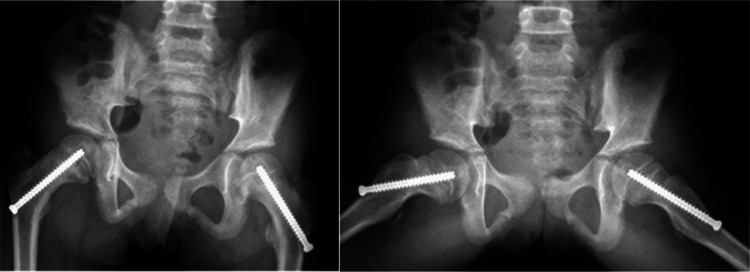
Anterioposterior and frog leg X-rays at the 10-month follow-up demonstrating complete epiphyseal migration and growth along cannulated screws.
